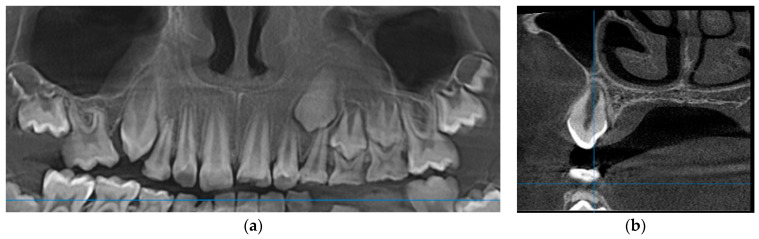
Case 1’s CBCT performed 9 months after the surgery: (a) panoramic view; (b) cross-sectional view.
